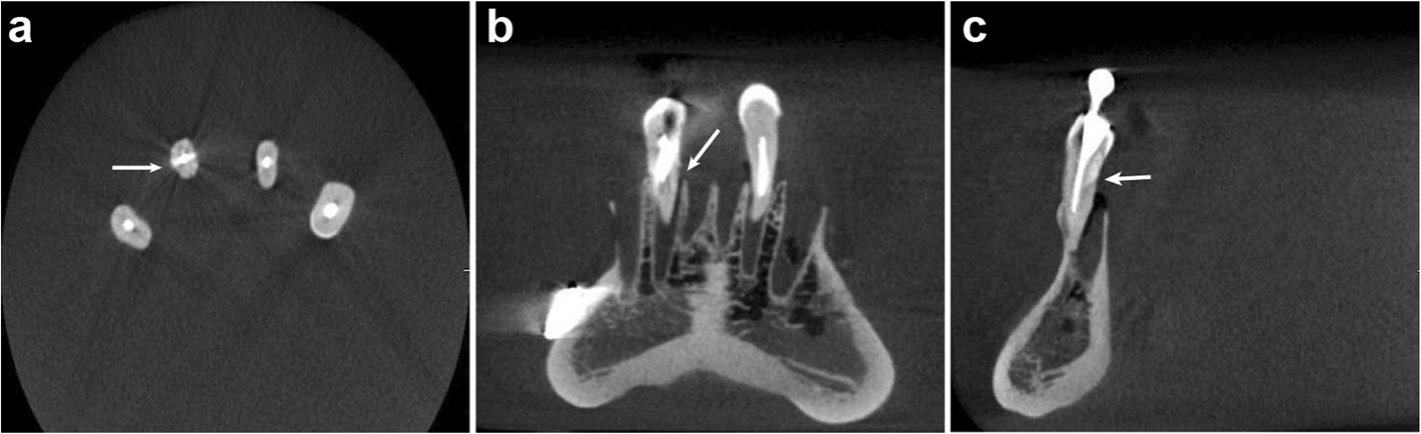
Prexion CBCT reconstructions. The vertical root fracture is observed in Axial (a), Coronal (b), and Sagital (c) slices in a tooth with a metal post
